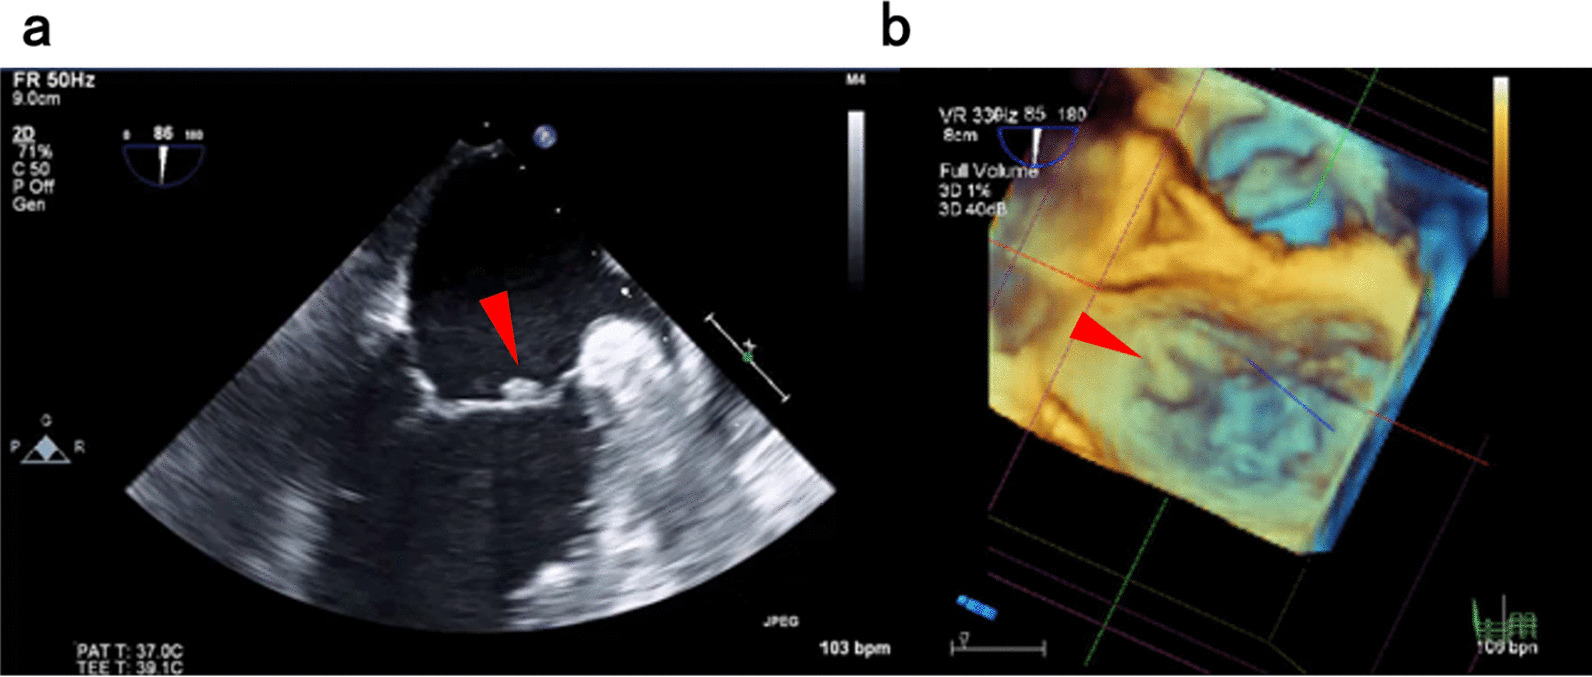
Transesophageal echocardiography images indicate (a) vegetation existence (arrowhead) and b prolapse of anterior commissure (arrowhead)
